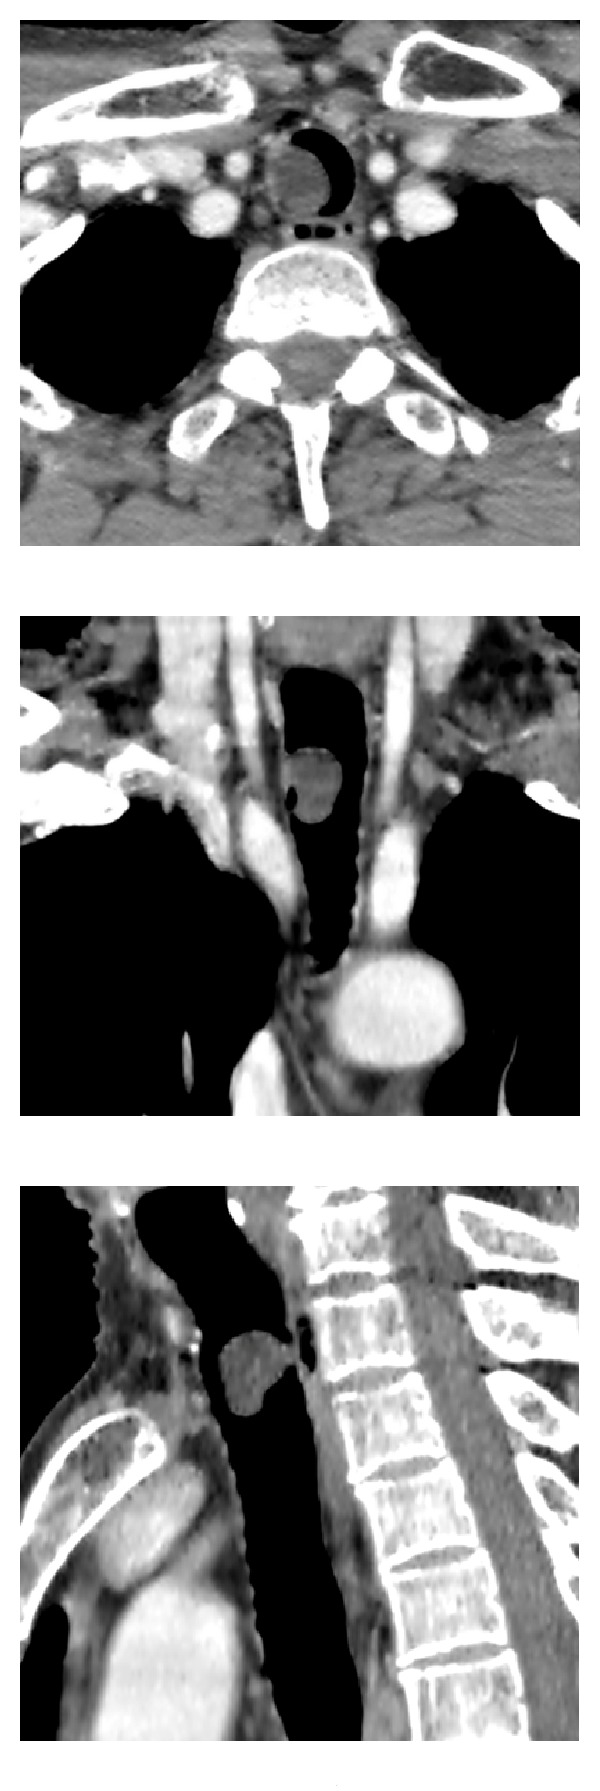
From left to right: axial, coronal, and sagittal CT views of the tumor located in the upper third of the trachea.
